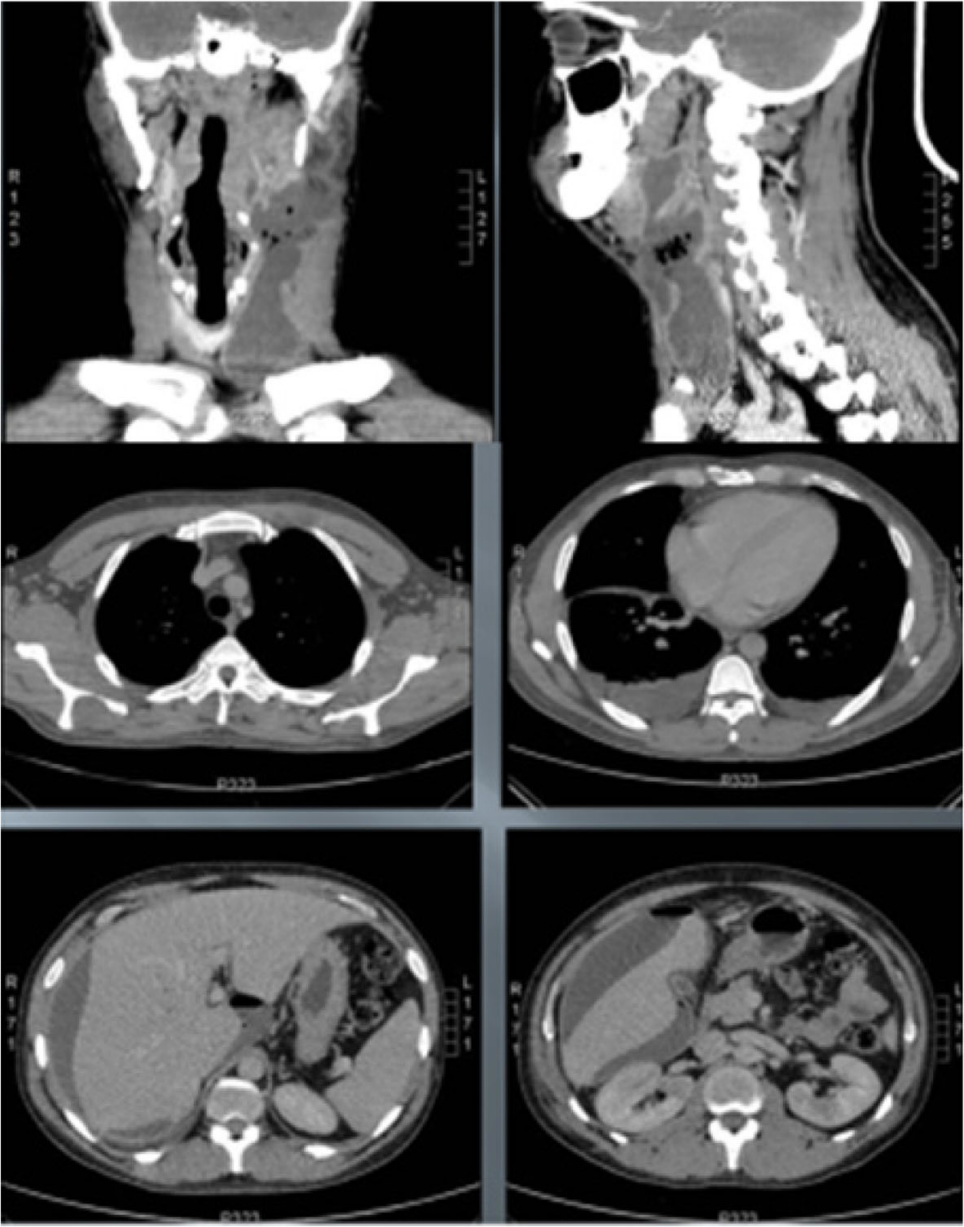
Computed tomography of the neck, thorax, and abdomen showing process extension
